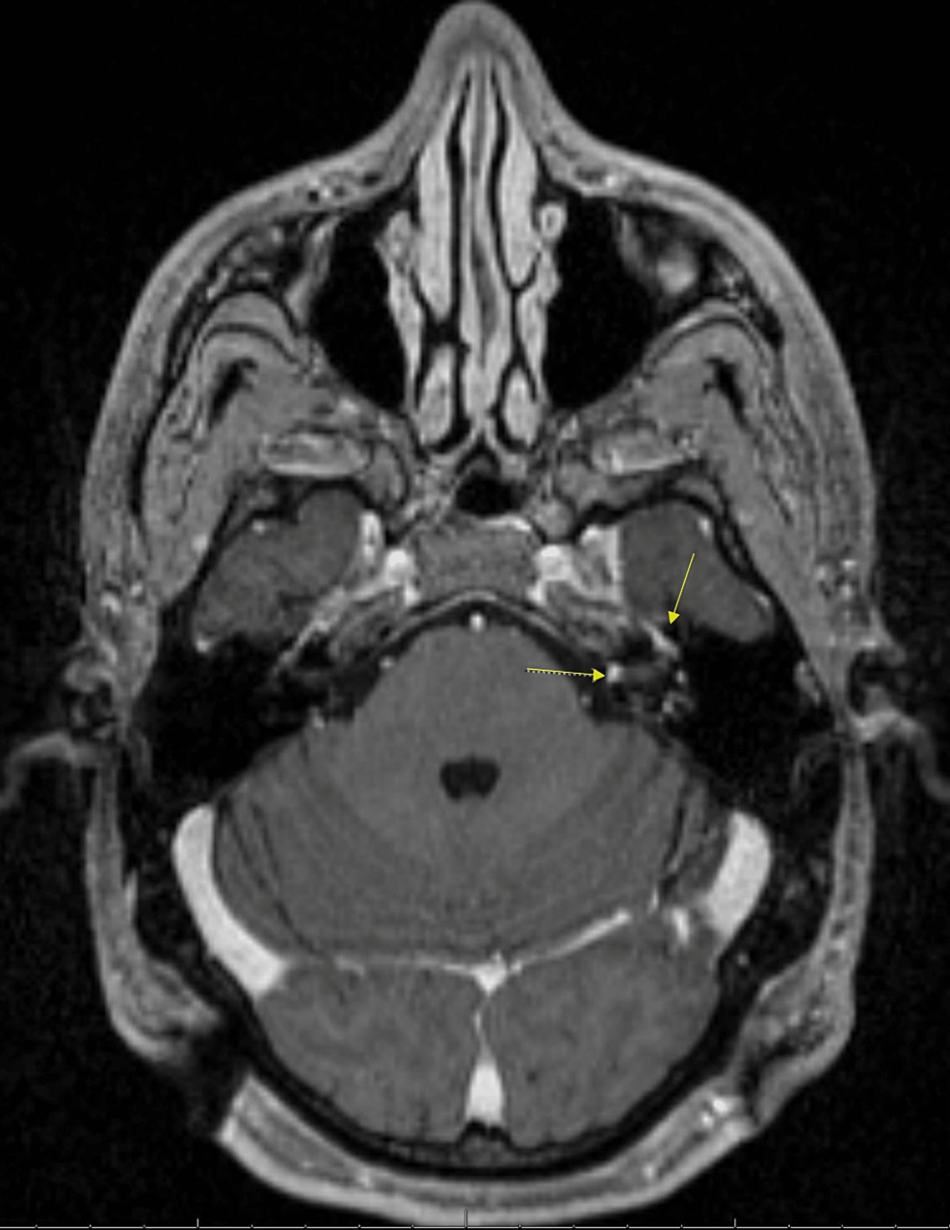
Axial T1 contrast MP RAGE sequence MRI of the brain, pre-gamma knife procedure four months after initial imaging The image shows residual enhancement in the left porus acusticus as well as the left apex of the cochlea shown by the arrows above.
